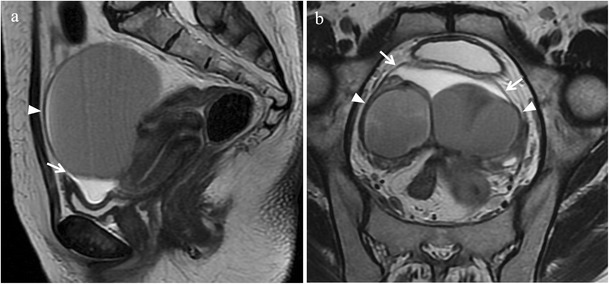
Indirect sign of adhesions in a 36-year-old woman with bilateral endometriomas. (a) Sagittal and (b) oblique coronal T2-weighted images show free fluid in the pelvis on “anti-declive position” (white arrows), due to the presence of adhesions and bilateral endometriomas (white arrowheads). Both ovaries are joined together involved in adhesions (kissing ovaries)
